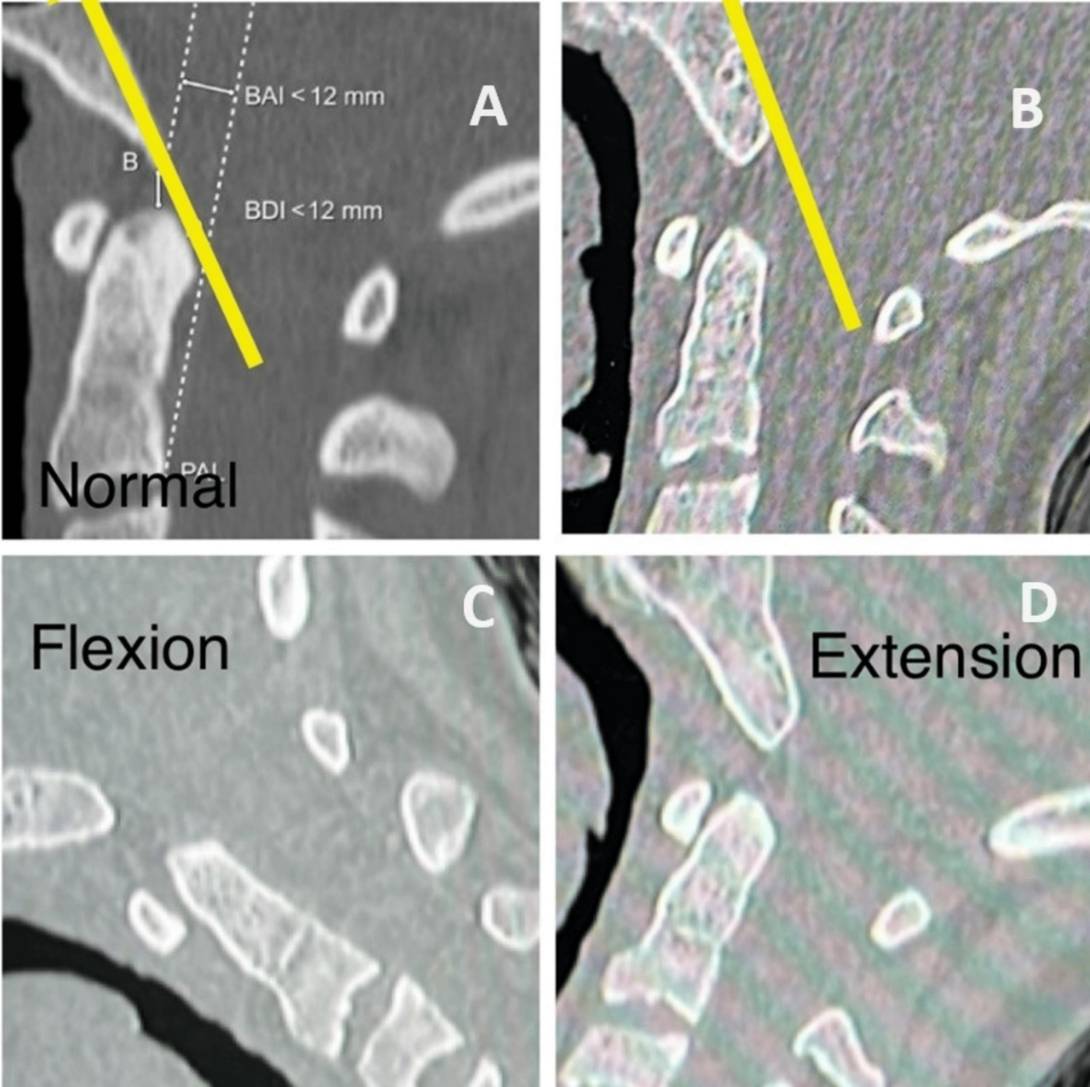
The patient’s CT scan showed a widening of the basion-dens interval (BDI) with vertical dissociation in neutral (B), flexion (C), and extension (D) views compared to normal one (A).
